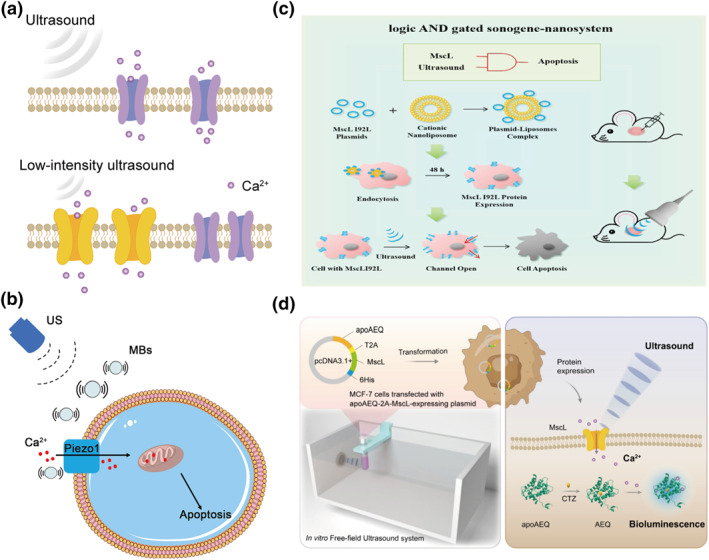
Sonogenetic strategies for cancer treatment. (a) Sonogenetic proteins decrease the celler response threshold to ultrasound. (b) Piezo1 expression in PDAC enables a noninvasive treatment strategy using US and MBs. Reproduced with permission. Reproduced with permission.^[56]^ Copyright 2022, Elsevier. (c) Logic AND‐gated sonogenetics for regulating tumor‐cell apoptosis. Reproduced with permission.^[57]^ Copyright 2020, American Chemical Society. (d) Proposed luminescence mechanism of a mechanoresponsive bioluminescent protein under ultrasound irradiation. Reproduced with permission. Reproduced with permission.^[58]^ Copyright 2025, American Chemical Society.
